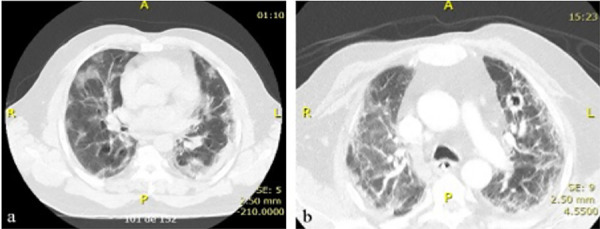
Computed tomography of the patient’s chest: (A) At hospital admission due to COVID-19; (B) At the time of isolation of *Sarocladium* spp. from the patient’s bloodstream.
